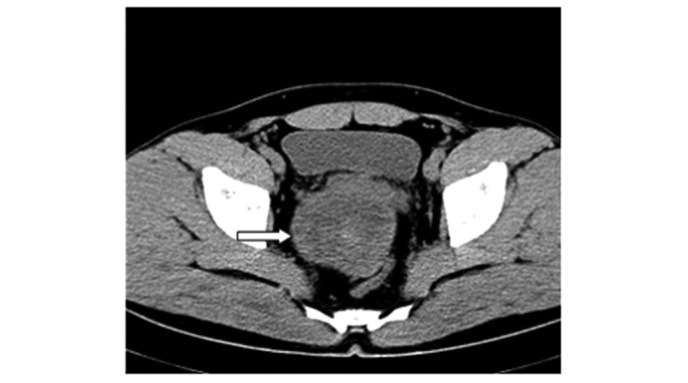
CT image demonstrating a 76×77×74-mm cystic mass and ring-like subcapsular high density district (arrows), without a connection with the adjacent tissues. CT, computed tomography.
